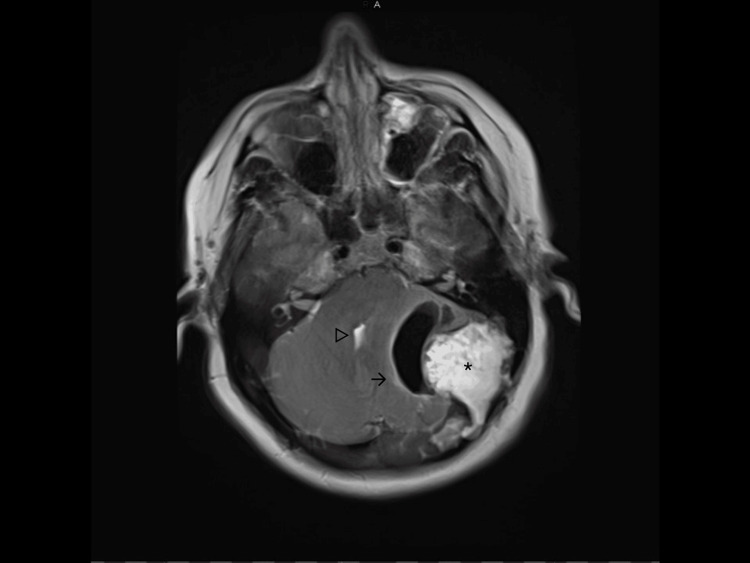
Preoperative MRI-T2 sequence showing a T2 hyperintense (asterisk) lesion surrounded with intracranial air (arrow) and mass effect compressing the fourth ventricle (triangle). MRI: Magnetic resonance imaging
